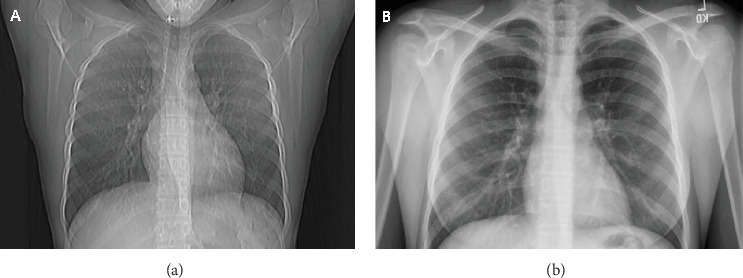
(a) Initial chest radiograph showing bilateral hazy airspace opacities. (b) Repeat chest radiograph following a 21-day steroid taper showing resolution of bilateral hazy airspace opacities.
